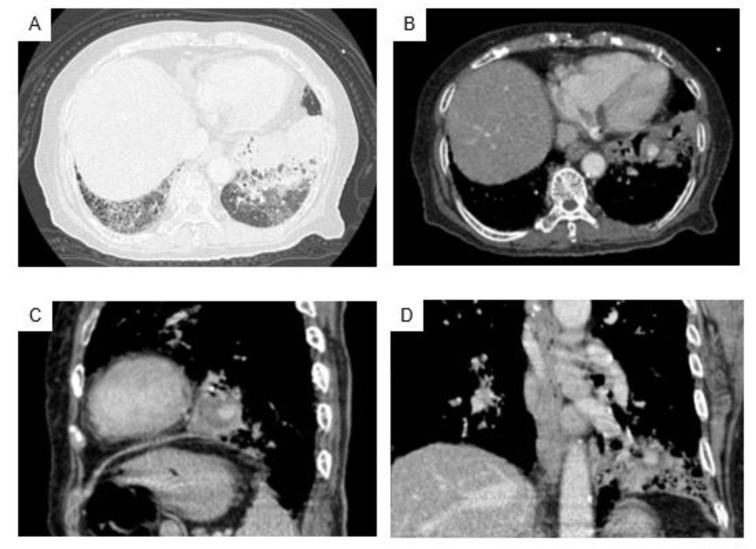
Contrast-enhanced chest CT on admission revealed grand-grass shadow and findings of honeycomb lung in bilateral lower lungs, and infiltrating shadow in the lower left lung field (A). An aneurysm with a contrast effect was found inside the infiltrated shadow in the left lower lung, without any obvious cavity lesion (B: axial view, C: sagittal view, D: coronal view).
